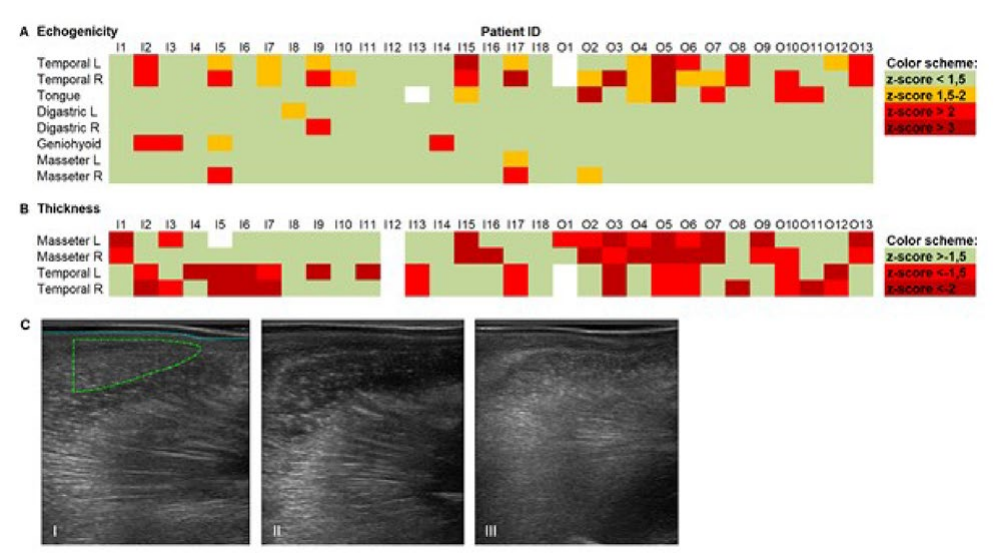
FIGURE 3 Quantitative muscle ultrasound (QMU) of orofacial muscles in IBM and OPMD patients.
